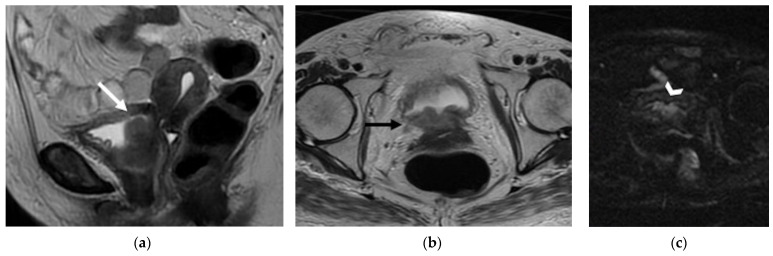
A 65-year-old woman who underwent chemoradiation therapy for cervical cancer. (a) Sagittal and axial (b) FSE T2-weighted image show an anterior pelvic recurrence, characterized by a soft-tissue mass infiltrating the posterior bladder wall extending into the bladder lumen (white and black arrow). (c) The lesion appears hyperintense on diffusion-weighted images (arrowhead).
